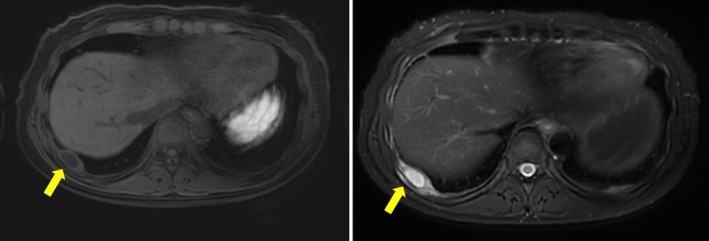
Magnetic resonance imaging (MRI) on presentation, demonstrating an almost 3‐cm tumour at the level of the 11th rib, which was low intensity by T1 and high intensity by T2. The tumour was marked with an arrow.
